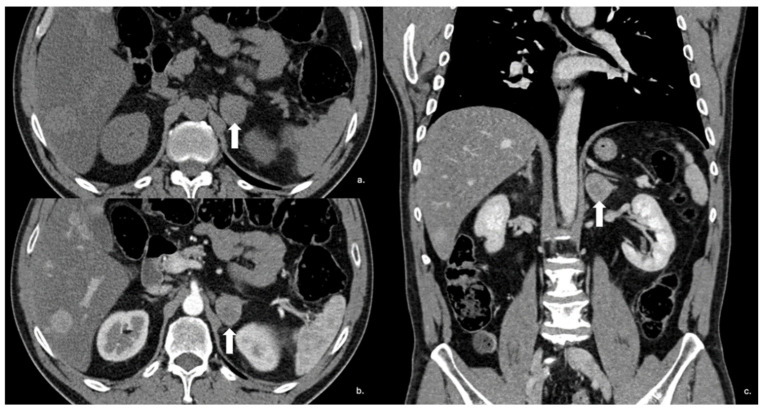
Axial unenhanced (a), arterial (b), and coronal venous (c) CT phases show a lung carcinoid metastasis (arrow) of the left adrenal gland. Additional hypervascular metastasis can be seen in the liver, particularly in the arterial phase.
